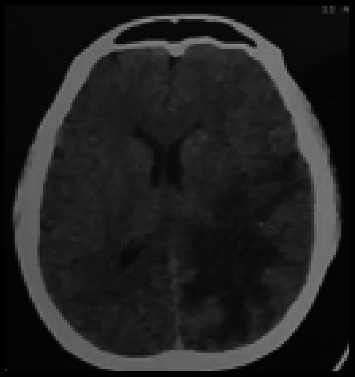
Computed tomography of the brain revealed a hypodense lesion in the left parieto-occipital region accompanied by marked perifocal edema.
